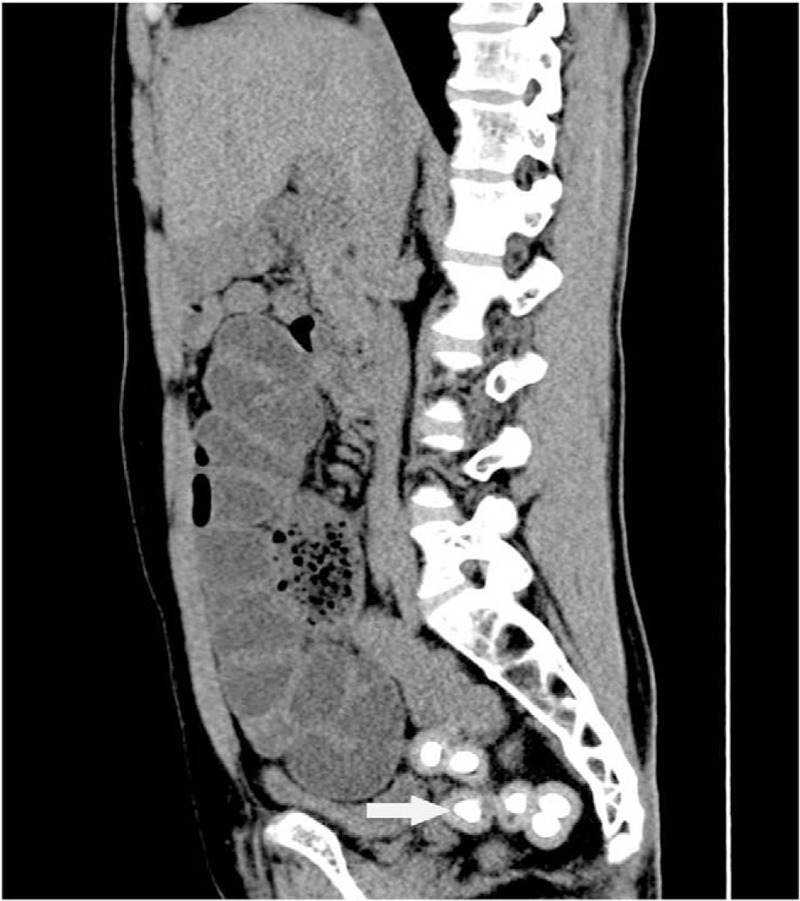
Abdominal coronal and sagittal CT images show multiple nodules in pelvic cavity, with central calcification and peripheral ring-like equidensite (arrow). Sagittal CT image shows dilated and tortuous intestine. CT = computed tomography.
